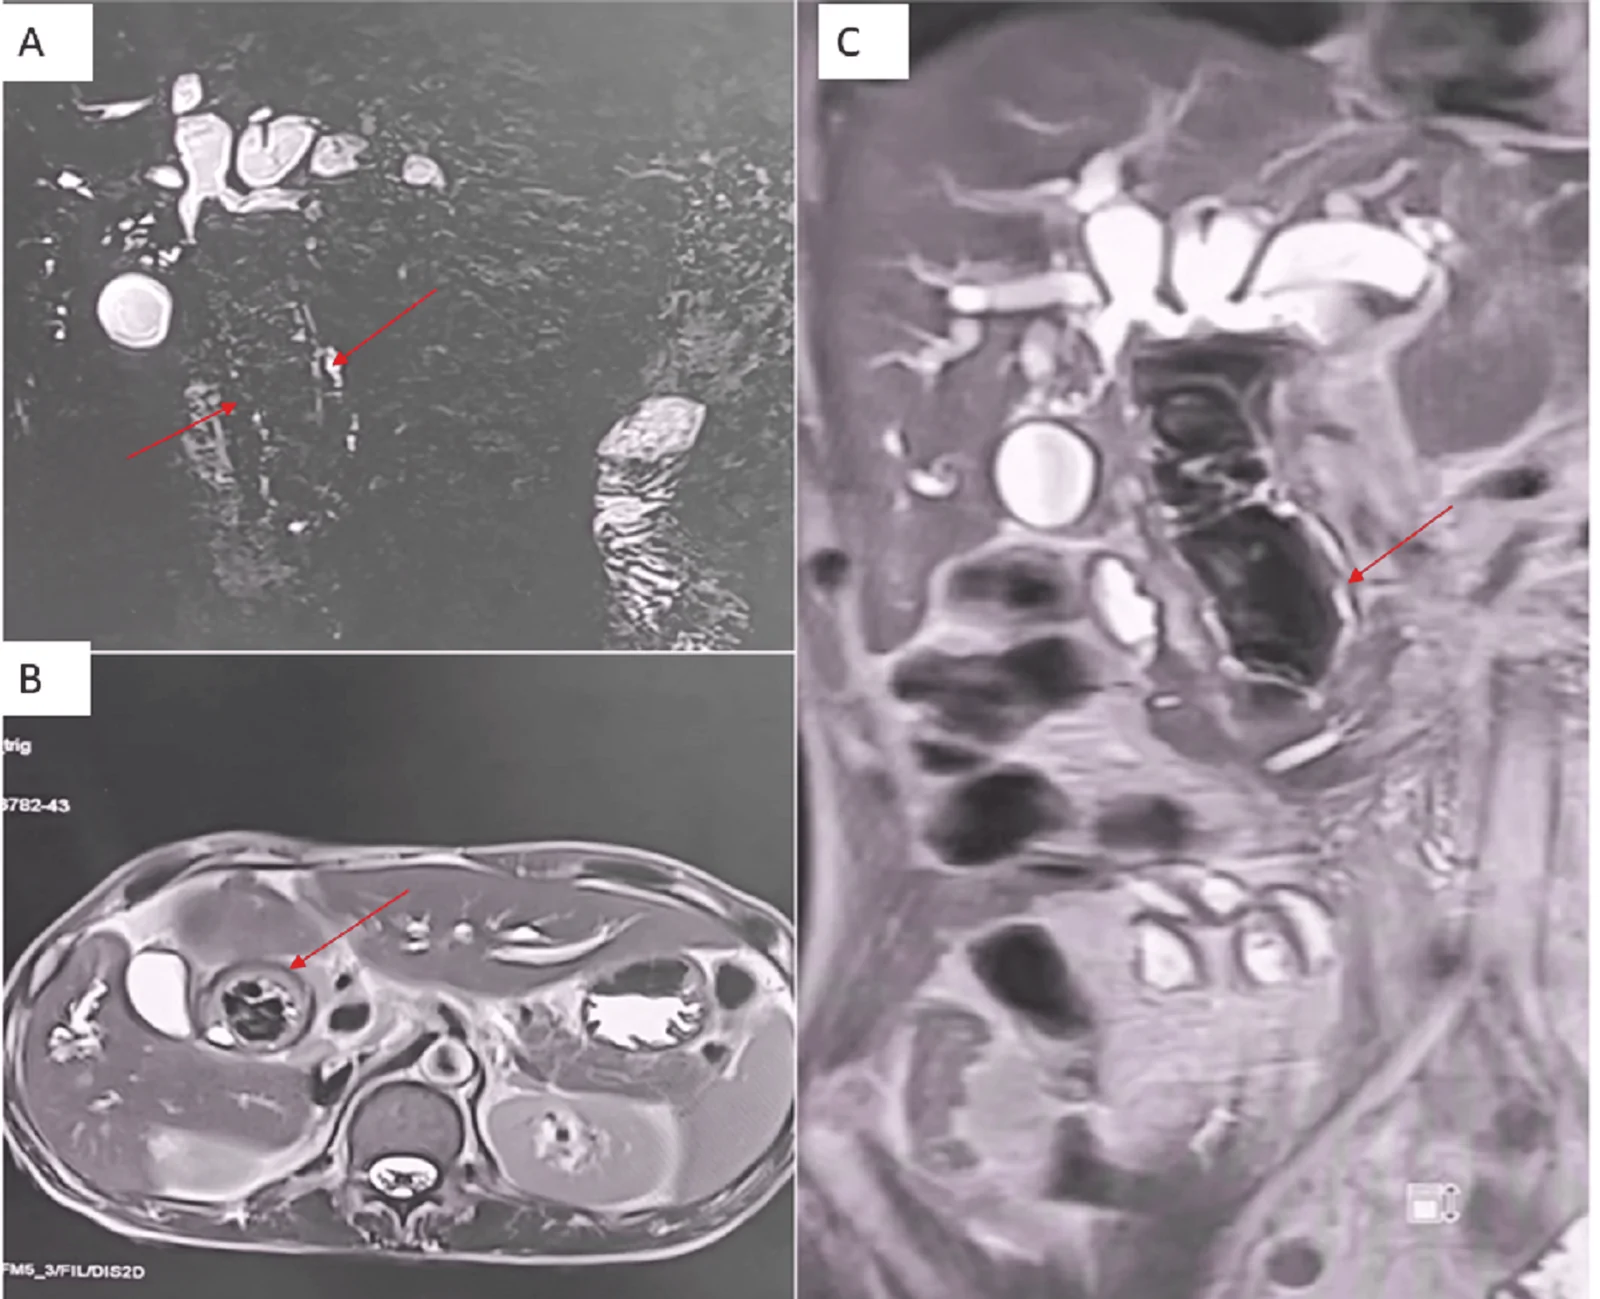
Magnetic resonance cholangiopancreatography (MRCP) images. (A) Heavily T2-weighted MRCP image showing a linear intraductal signal void within the common bile duct (red arrows) with surrounding intraductal filling defect. (B) Axial T2-weighted image demonstrating a rounded hypointense intraluminal lesion within a dilated CBD (red arrow). (C) Coronal MRCP image showing CBD dilatation with a central linear signal void and adherent filling defect (red arrow), causing biliary obstruction. CBD: common bile duct
